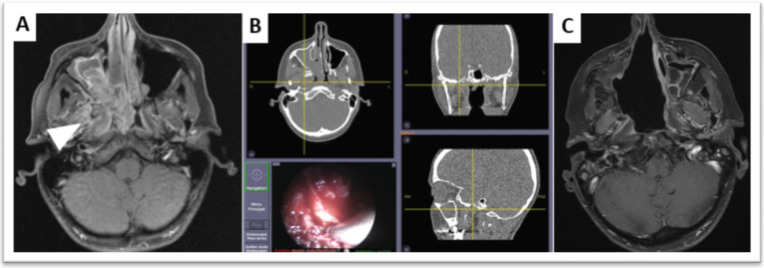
Surgical excision of an adenoid cystic carcinoma of the right nasal fossa using a surgical navigation system. (A): pre-operative contrast-enhanced T1-weighted MRI displays a right nasal fossa tumor invading the root of the pterygoids and the pterygoid muscles (arrowhead). (B): intraoperative computer-assisted surgical navigation system (DigiPointeur^®^, Collin, France) helps to correlate endoscopic and CT visualization of the tumor extension within the pterygoid muscles. (C): contrast-enhanced T1-weighted MRI confirms that the patient is free of local recurrence after 14 years of follow-up.
